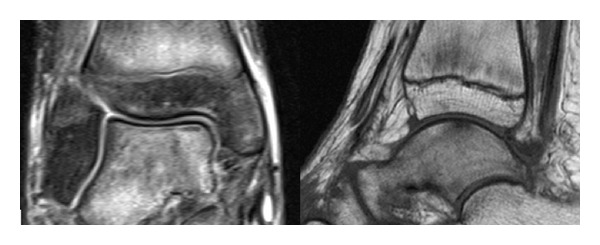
A coronal fat suppressed T2-weighted image and a sagittal T1-weighted image of a 12-year-old girl after ankle sprain showing diffuse bone marrow edema of the talus and the tibia. On conventional imaging, only subchondral bandlike osteoporosis of the talar dome could be seen (Figure 2).
